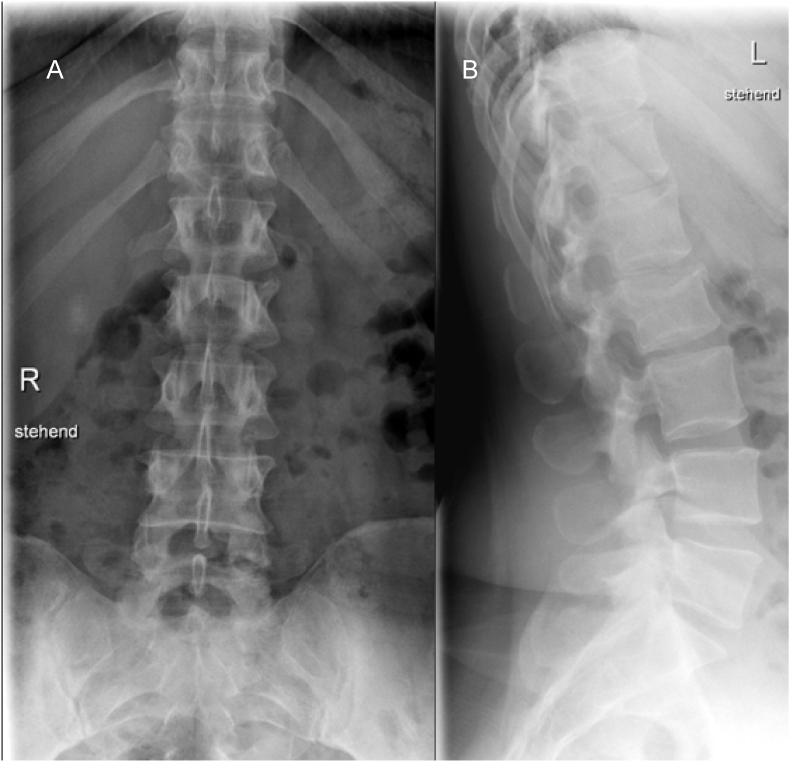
X-ray of the lumbar spine (AP and lateral) after the trauma. An endplate compression fracture without the posterior edge involvement is shown in the lateral image.
